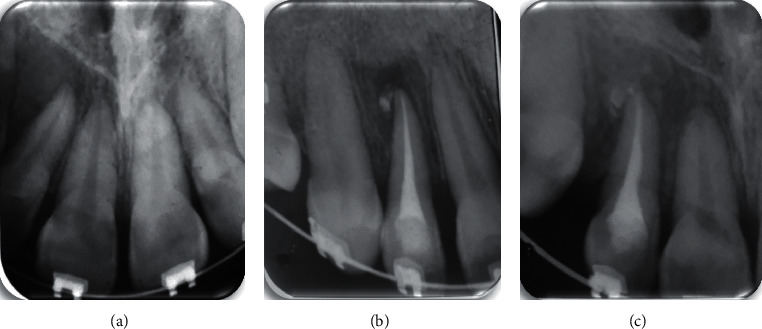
A case of a healing lesion: (a) preoperative radiograph; (b) postoperative radiograph showing the extrusion of Bioroot RCS; and (c) a 6-month follow-up radiograph.
